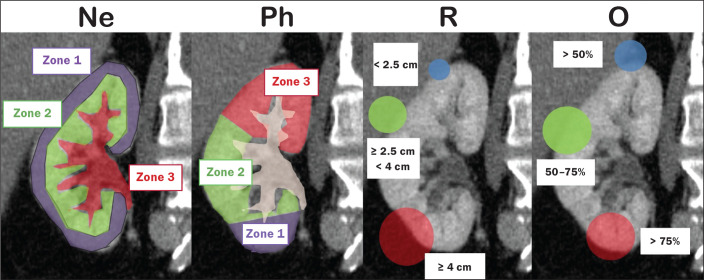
Sagittal CT scan of the kidney showing the renal zones and tumor characteristics used in order to calculate risk with the NePhRO score. Ne, proximity to the renal collecting system; Ph, physical zones; R, radius of the mass; O, organization of the mass; Zone 1, the lower pole, below the renal collecting system; Zone 2, lateral to the collecting system without touching it and excluding the upper pole; Zone 3, the upper pole, the collecting system, and all components of the hilum.
